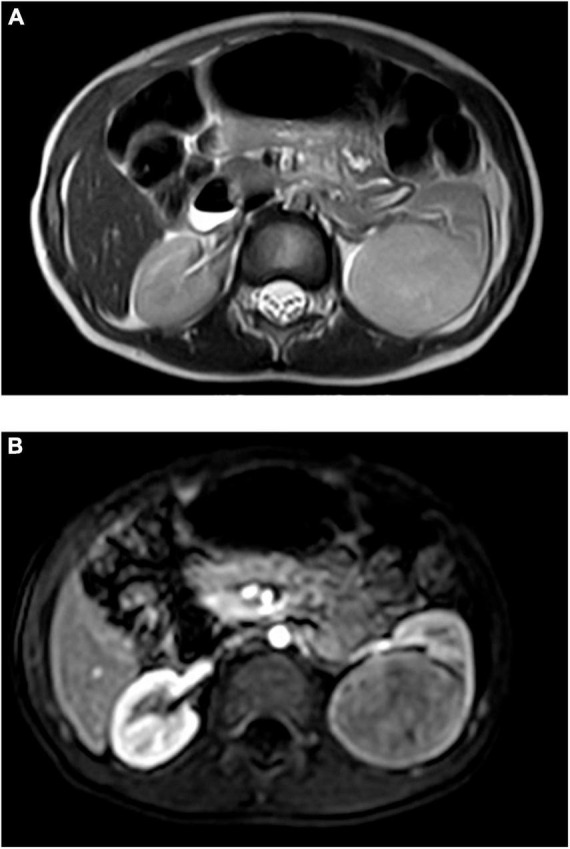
(A) MRI plain scan before chemotherapy: equisignal on T1WI, slightly higher signal on T2WI, significantly higher signal on DWI, and low signal on ADC. (B) MRI enhancement before chemotherapy: dynamic enhancement showed delayed uneven enhancement, and the degree was weaker than that of renal cortex.
